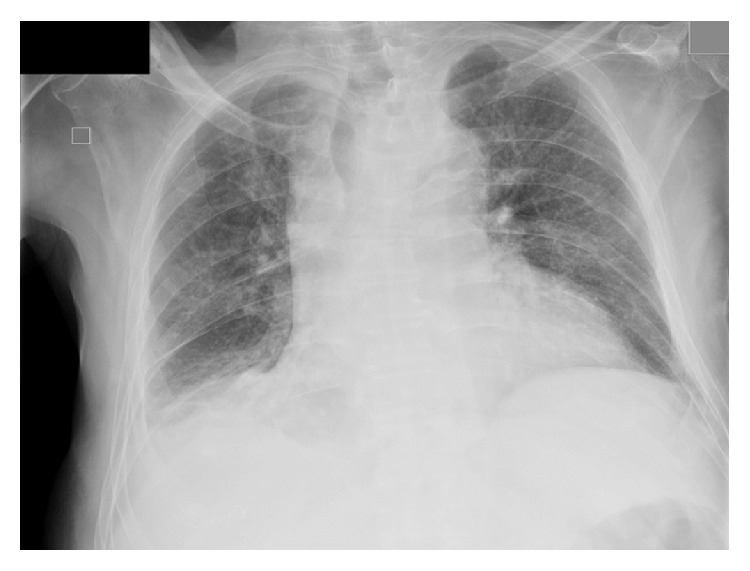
Control chest X-ray on the follow-up visit. Radiological improvement of the right lower lobe alveolar infiltrate.
